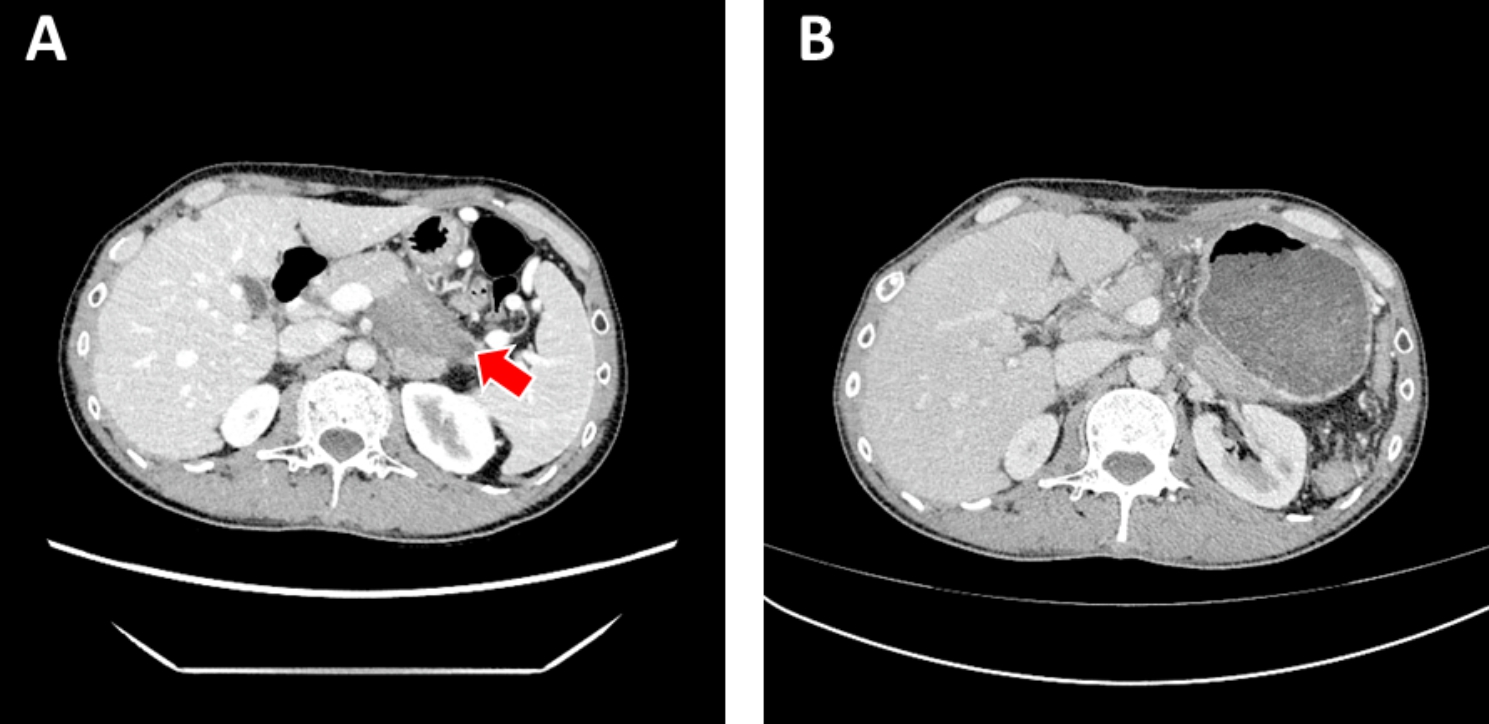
CT scan of the abdomen Hypodense mass (arrow) in the body and tail of the pancreas at diagnosis (A); The absence of body and tail of the pancreas, left adrenal gland and spleen after operation, and a small amount of effusion in the original operation area is basically absorbed after 3 courses of chemotherapy with CAV/IE regimen (B)
